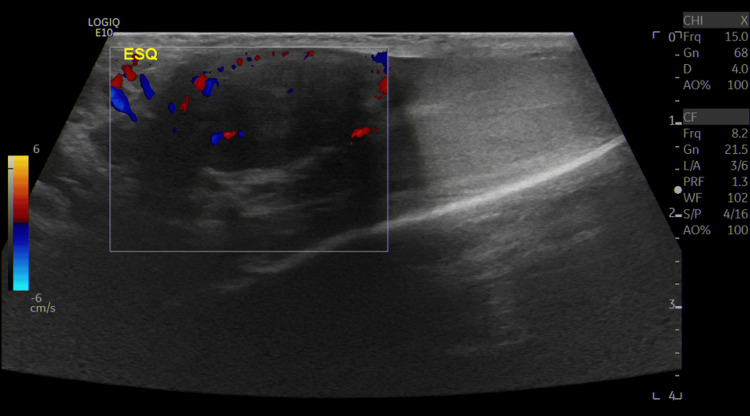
Scrotal ultrasonography showing heterogeneous and infiltrative left paratesticular mass, with positive color flow Doppler.
